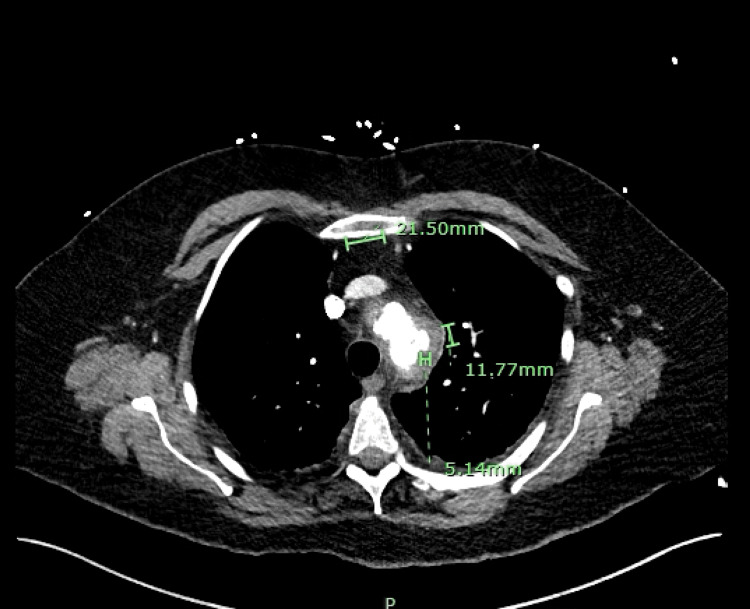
CT Angiogram Chest showing 0.5 x 1.2 cm eccentric hyperdense collection outside the boundary of the aorta
